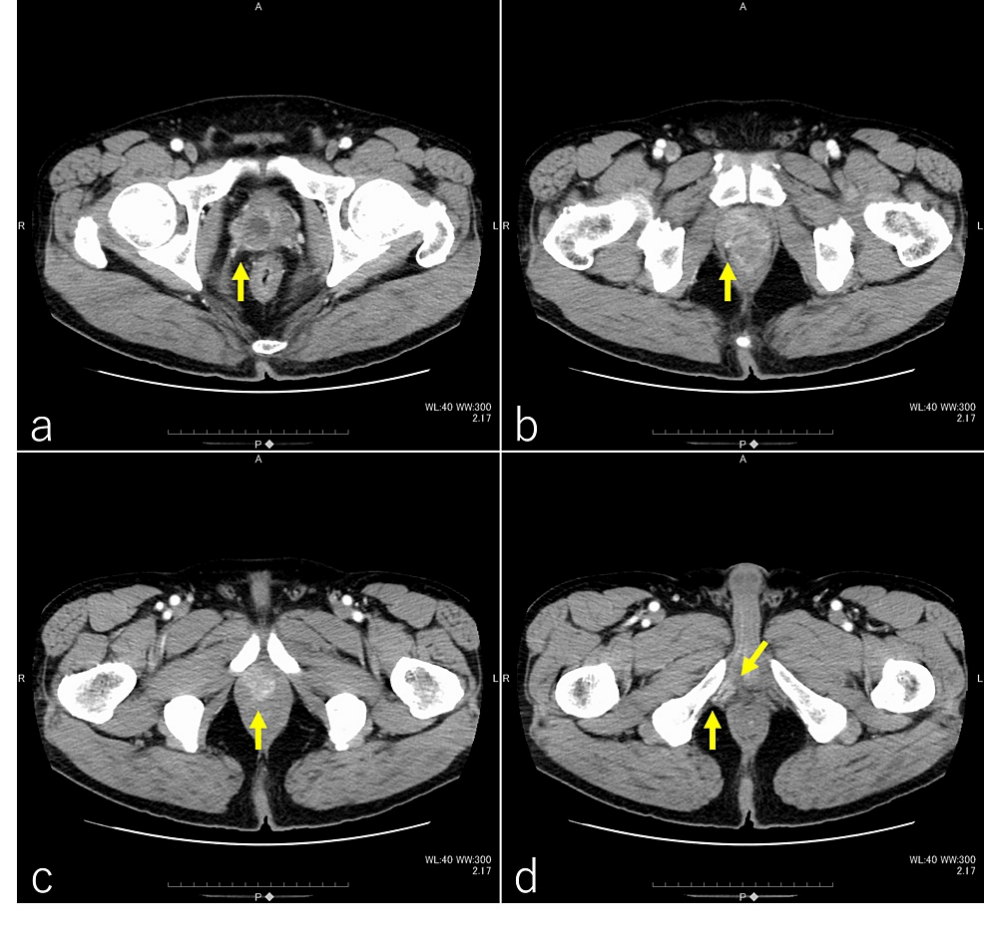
Pelvic contrast-enhanced CT The prostate is predominantly enlarged on the right side, with a visible mass displaying a contrast effect (indicated by arrows in a and b). There is involvement extending toward the apex of the prostate (indicated by an arrow in c) and invasion into the ischiocavernosus muscle and the superficial transverse perineal muscle (indicated by arrows in d).
